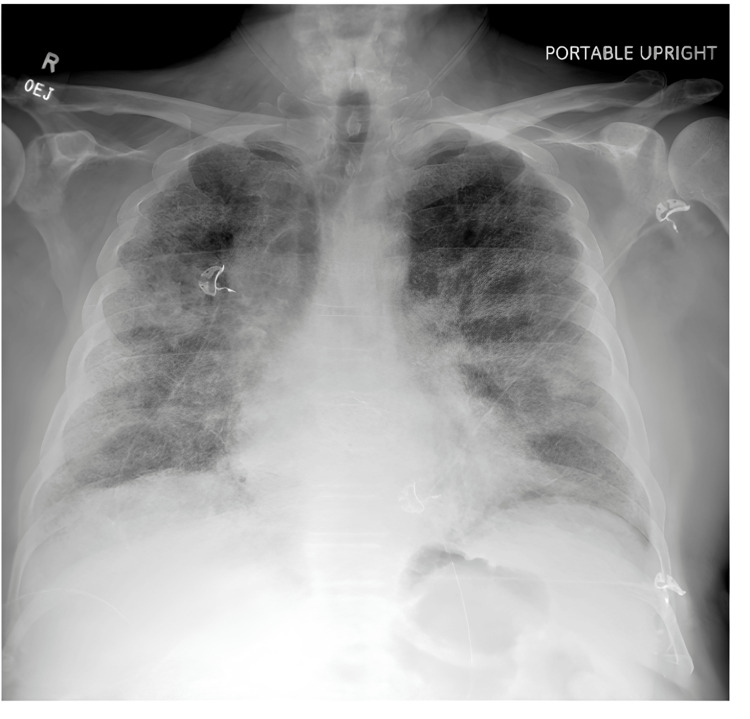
Chest radiograph demonstrating diffuse bilateral increased interstitial lung markings, most consistent with diffuse interstitial infiltrates
